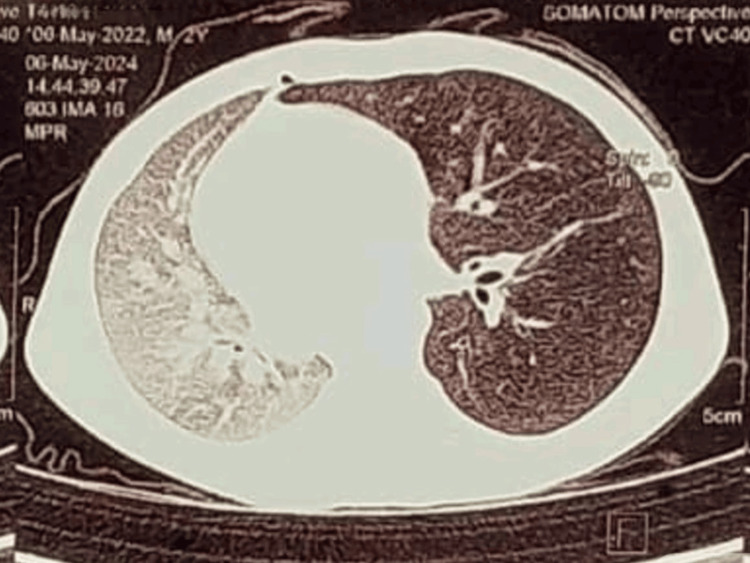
Contrast-enhanced CT thorax, axial view Window for pulmonary parenchyma in axial view showing overinflation of the left lung and midline herniation, accompanied by a contralateral tracheal and mediastinal shift.
